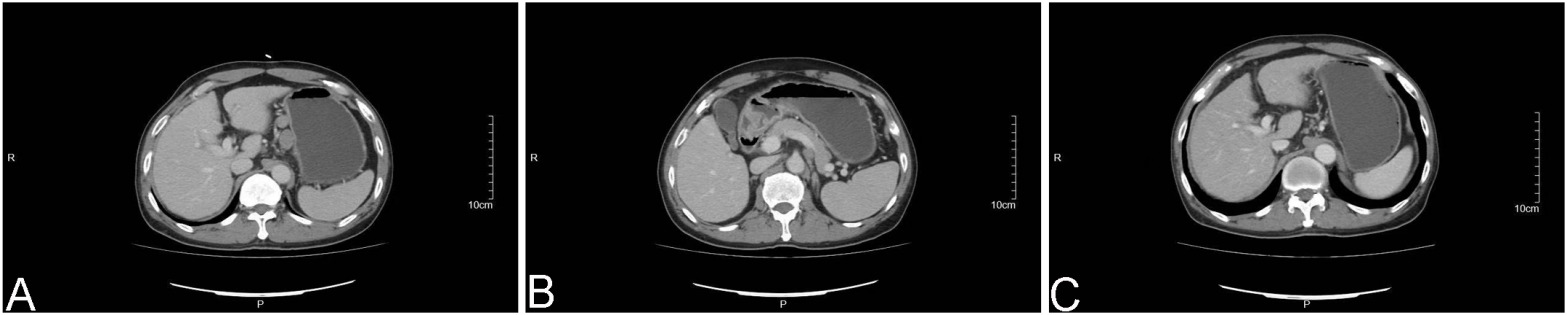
Computed tomography (CT) images: (A) An abdominal CT scan from August 2013 reveals enlarged lymph nodes adjacent to the lesser curvature of the stomach; (B) An abdominal CT scan from August 2013 shows enlarged lymph nodes in the retroperitoneal area, with no space-occupying lesion identified in the gastric wall; (C) An abdominal CT scan from February 2016 demonstrates no evidence of enlarged lymph nodes.
